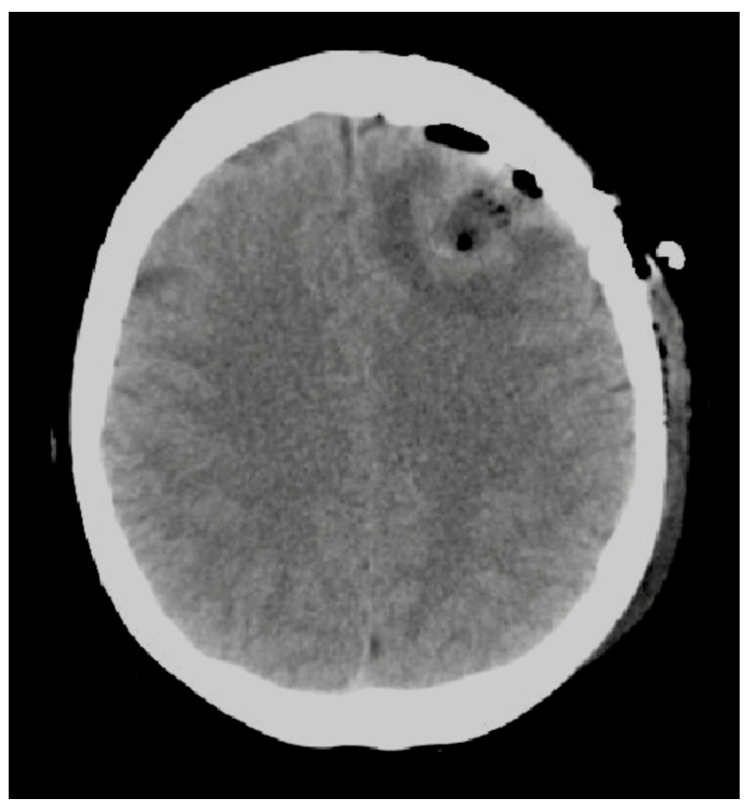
Shows a postoperative CT scan confirming the absence of surgical complications.
